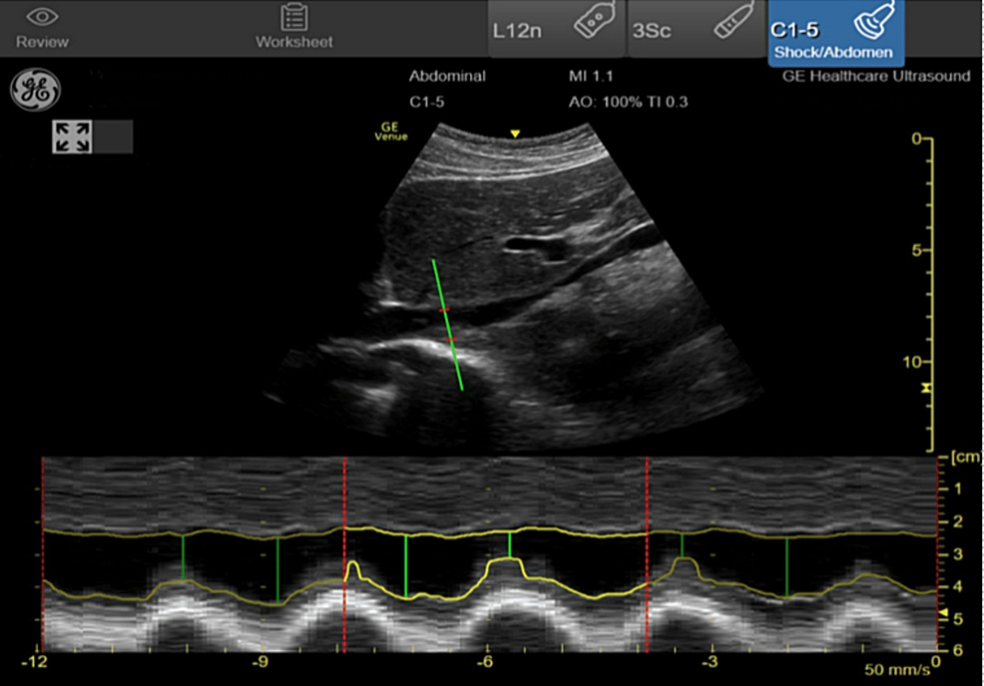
Point of care ultrasound with semi-automated software platform with IVC IVC: inferior vena cava
